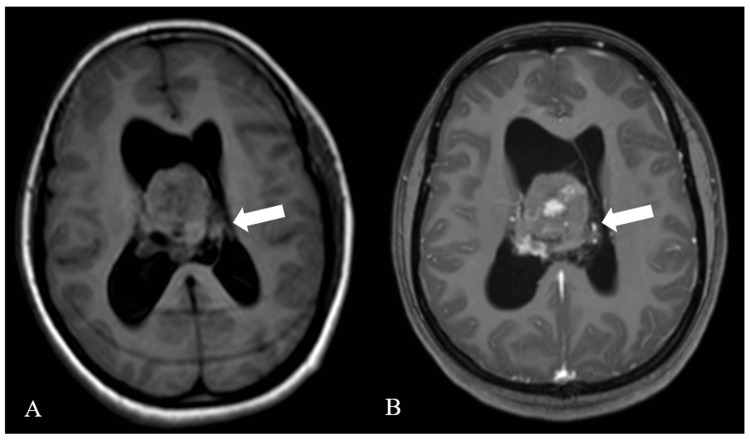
T1-weighted MRI. T1WI axial (A) and T1 contrast axial (B) MRI: Magnetic resonance imaging; T1WI: T1-weighted image
